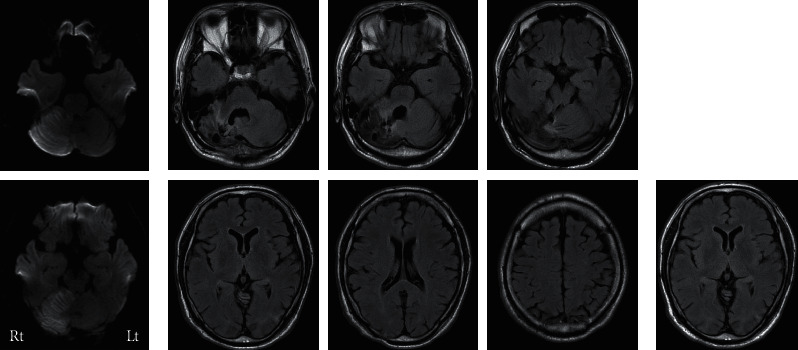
(a) Magnetic resonance (MR) images on day 0. Axial diffusion weighted images demonstrating a very new infarction in the right cerebellar hemisphere. Rt: right, Lt: left. (b) MR images on day 148. Axial FLAIR (fluid attenuated inversion recovery) MR images demonstrating no evidence of supratentorial damage without current infarction in the right cerebellar hemisphere. (c) A MR image 3 years after stroke onset. An axial FLAIR MR image demonstrating still no structural damage on the left posterior middle temporal gyrus.
